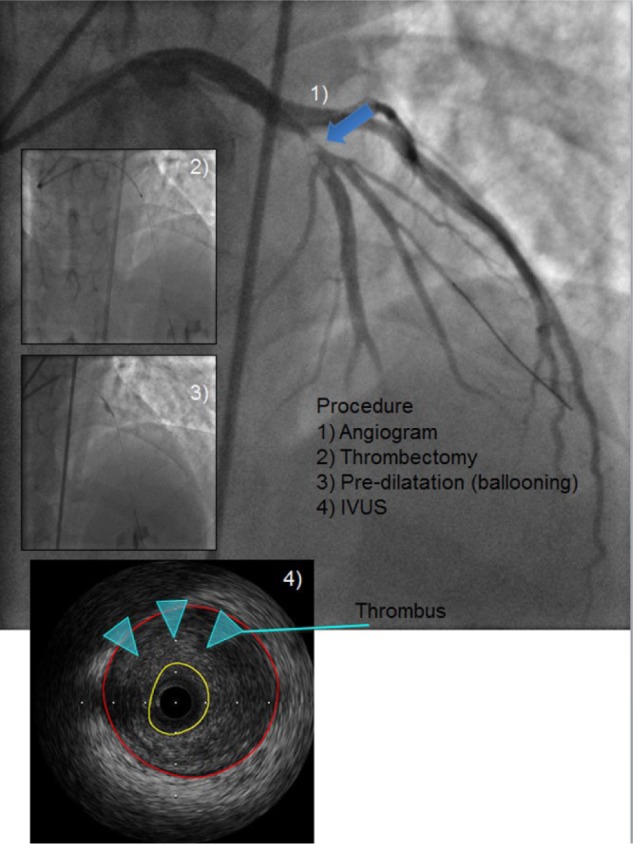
Coronary angiogram (1) showing a hazy lesion in the proximal LAD (single blue arrow). Following thrombectomy (2) and predilatation with balloon angioplasty (3), IVUS demonstrated a thrombus (turquoise arrow heads) in the culprit lesion (4). LAD, left anterior descending artery; IVUS, intravascular ultrasound.
